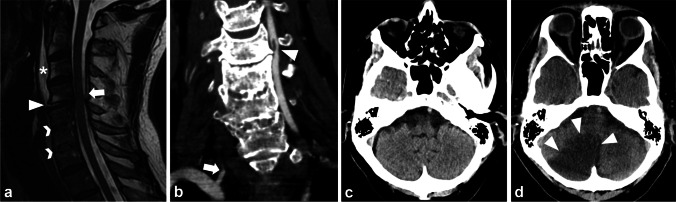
A 67-year-old patient with ankylosis from DS between the fifth and seventh cervical vertebrae after two anterior intervertebral disc implants between the fifth and sixth and the sixth and seventh cervical vertebrae suffered an impact from being struck by heavy construction scaffolding, resulting in an unstable fracture through the intervertebral disc between the fourth and fifth cervical vertebrae (arrowhead). T2w MRI in the sagittal plane reveals posterior epidural hematoma (arrow) and prevertebral hemorrhage (asterisk) (a). This CTA coronal image with 2 mm slice thickness shows a grade II BCVI with an intraluminal thrombus located in the left VA (arrowhead), while the right VA is occluded owing to a grade IV BCVI (arrow) (b). An unenhanced head CT taken in the acute phase showed no signs of acute ischemia (transverse reformat with 3 mm slice thickness (c). Two days after the initial injury, a cerebellar infarction is demarcated as a clearly hypodense area in transverse reformat with 3 mm slice thickness (d). BCVI, blunt cerebrovascular injury; CTA, CT angiography
